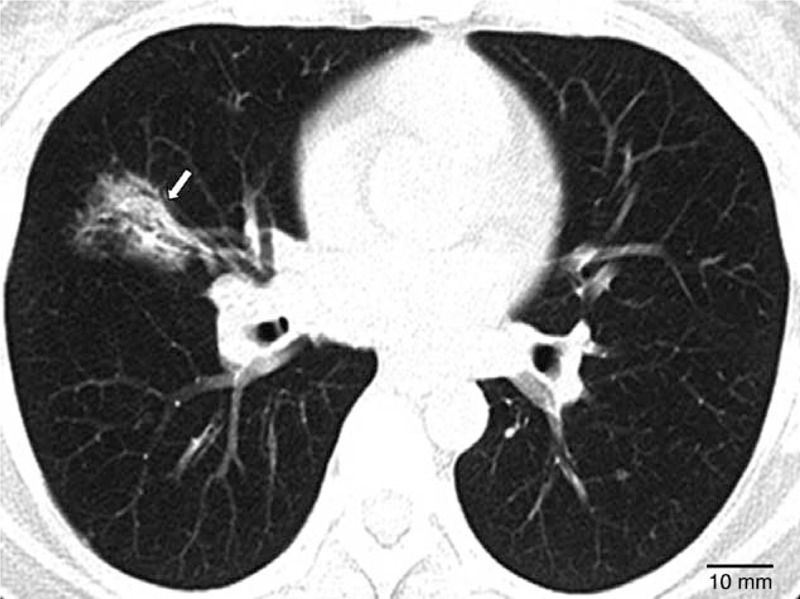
CT images showing pneumonia-like consolidation (homogenous opacity) characterized by little or no loss of volume, effacement of blood-vessel shadows, and presence of an air bronchogram. CT = computed tomography.
